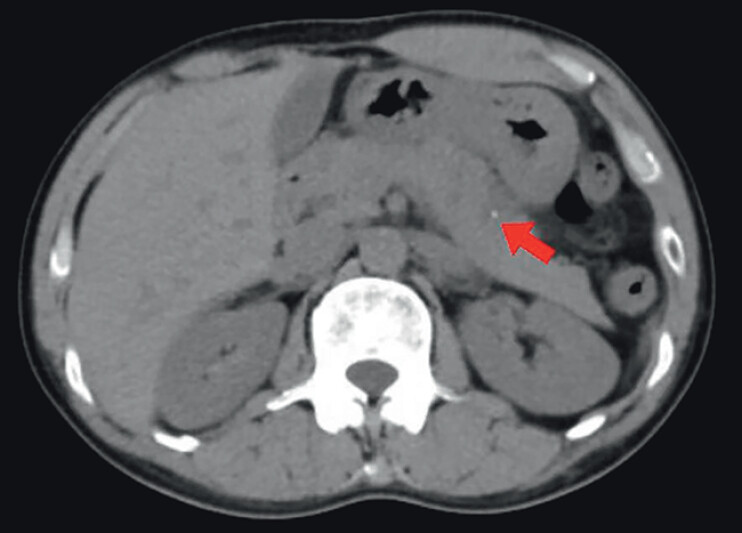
Abdominal computed tomography image showing a thin strip of high density shadowing on the lower curved side of the stomach body and the pancreatic body.
